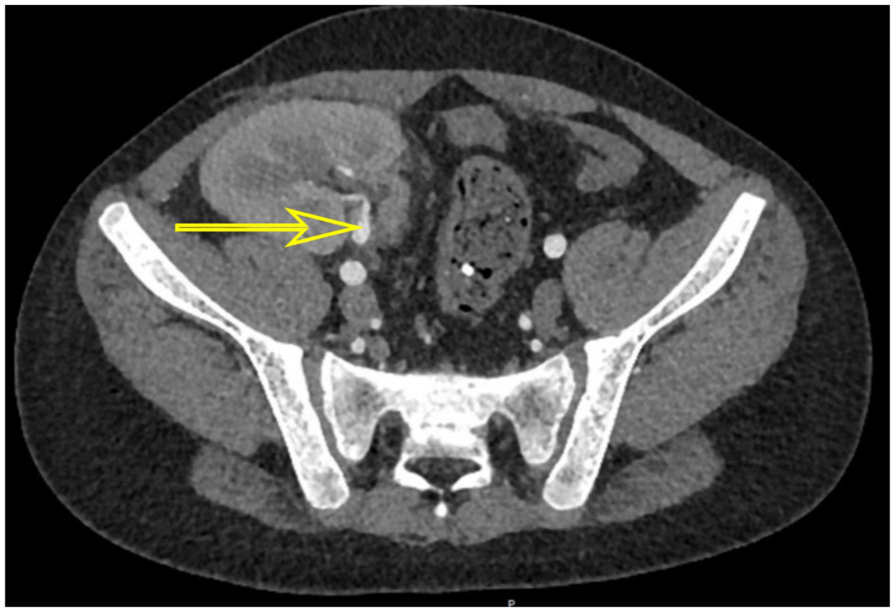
CT scan showed transplanted renal artery stenosis (yellow arrow).
